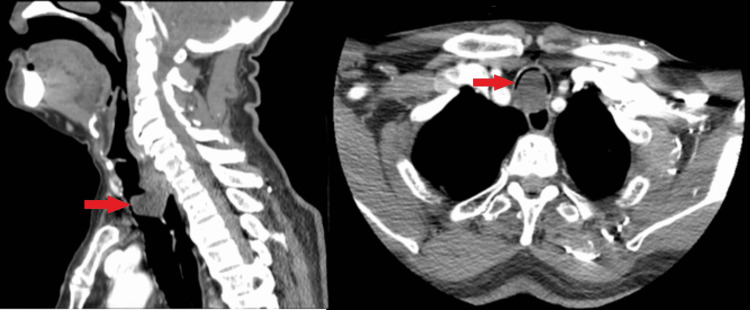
Sagittal and axial computed tomography images of near obstructing tracheal mass
